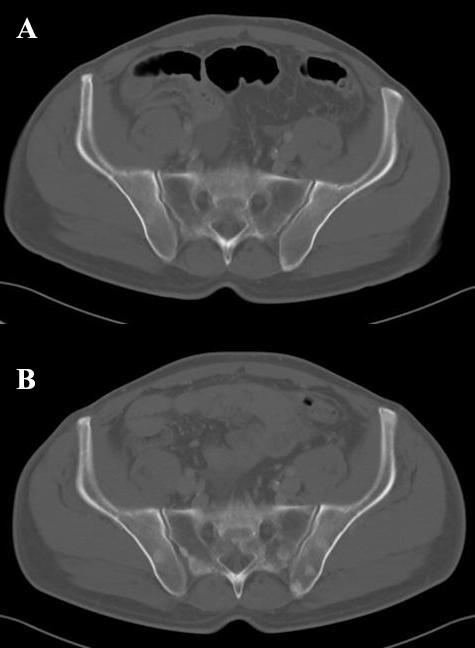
A representative bone window scan of the pelvis. In A, the bone window scan of the pelvis at baseline, before the administration of chemotherapy is shown. No clear osseous metastasis are seen. In B, the CT scan of the same region after two cycles of pemetrexed and oxaliplatin shows diffuse osteoblastic lesions which are apparent in the sacrum and iliac bones.
